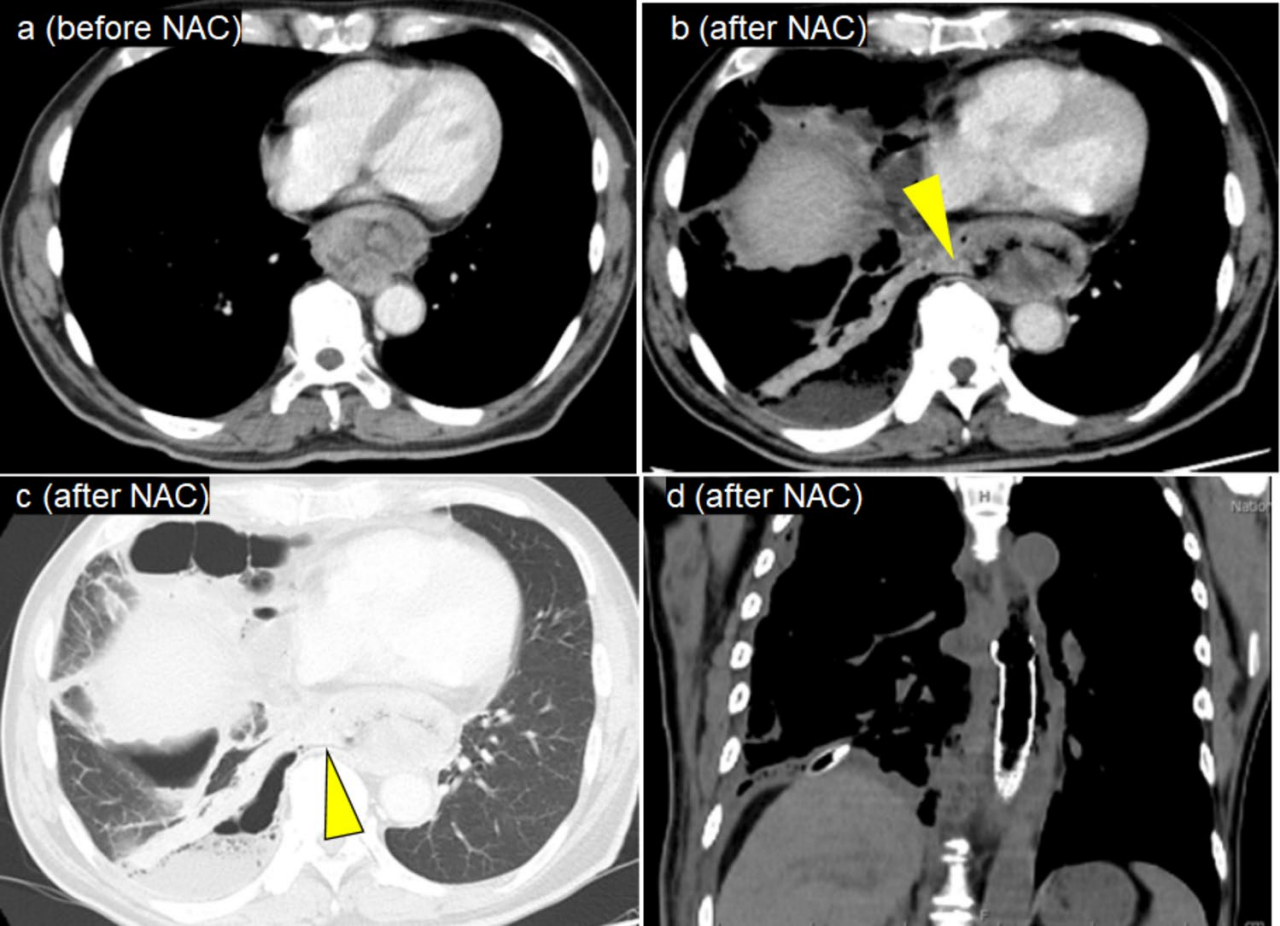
Clinical course images of Case 3. (a) Contrast-enhanced CT scan showing a tumor in the middle thoracic esophagus. (b, c) CT scans showing right-sided pneumothorax, pyothorax, and an esophagorespiratory fistula (arrowhead). (d) CT image showing esophageal stent and drainage for right-sided pyothorax. NAC: neoadjuvant chemotherapy
